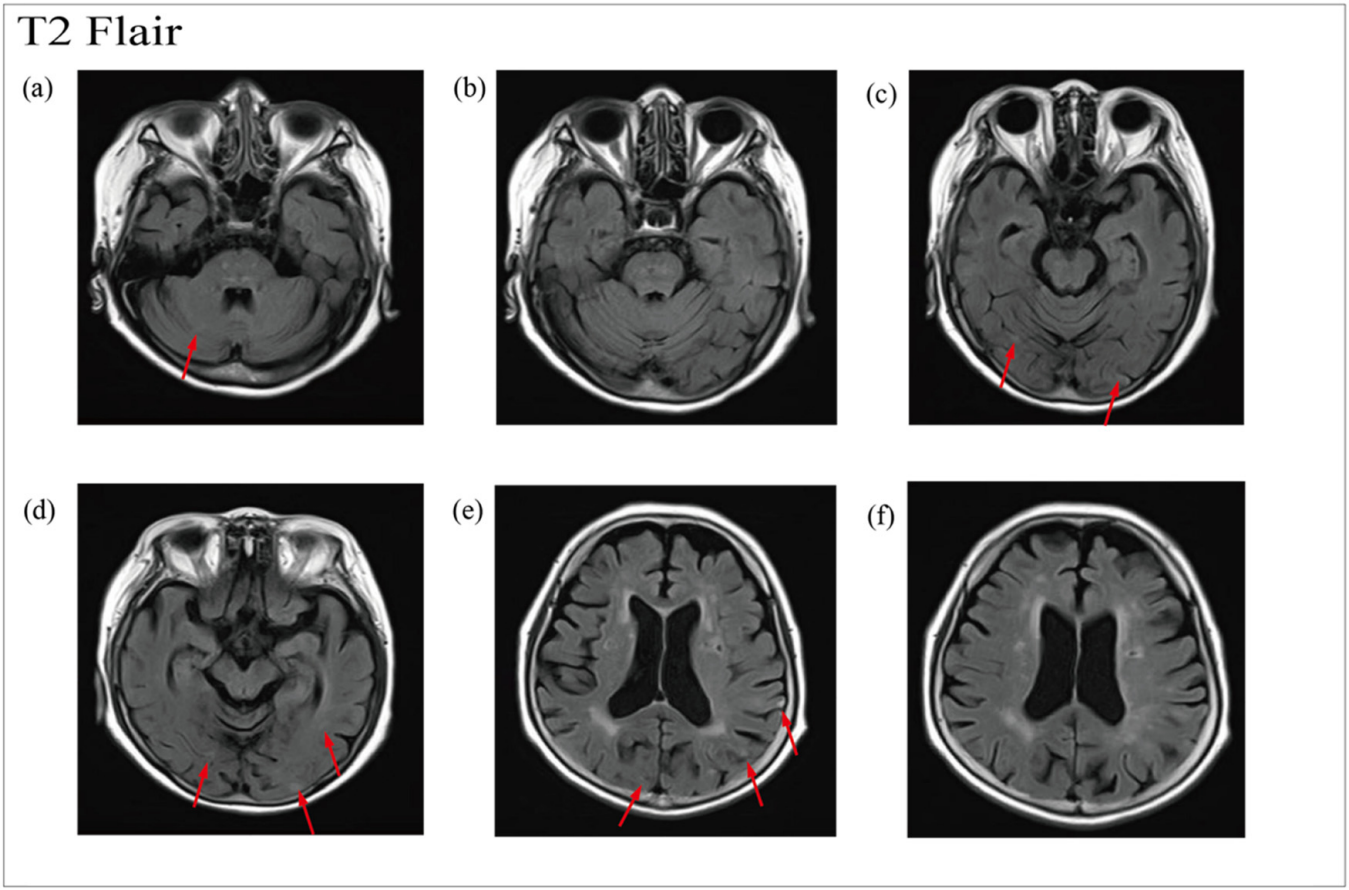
T2 Flair sequence of cerebral MRI. (a–f) Multiple lacunar infarcts in cerebral cortex, subcortex and cerebellum.
